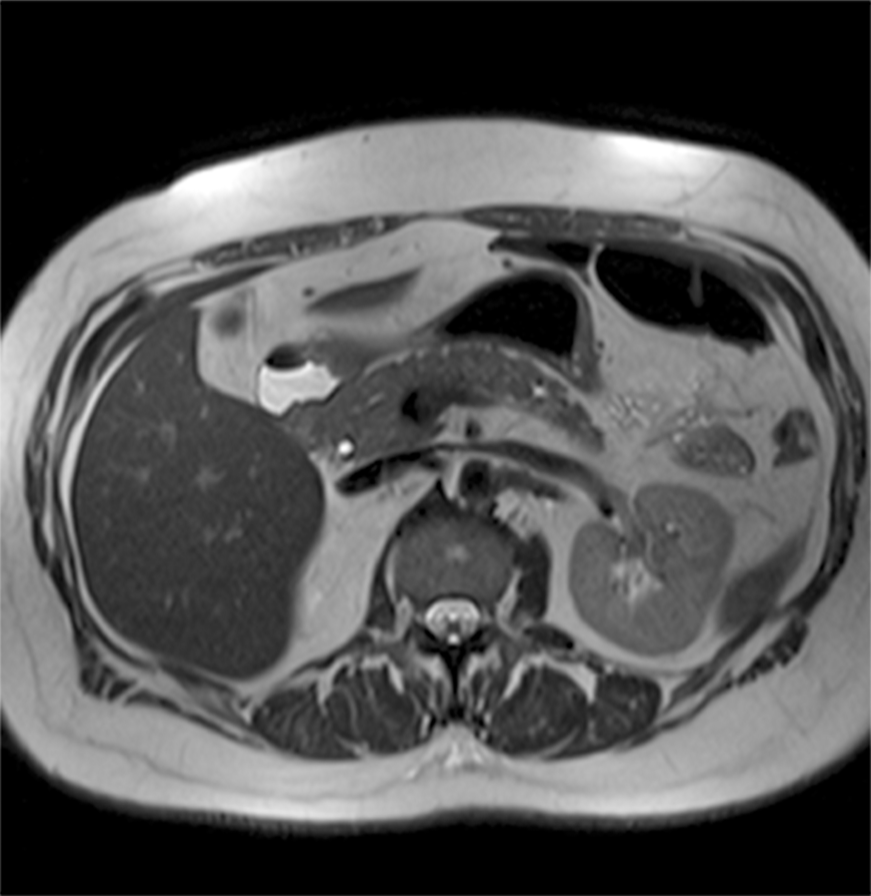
Abdominal MRI (T2-weighted sequence, axial slice), showing an additional small cyst located at the transition between body and tail of pancreas. Same patient as Fig. 2.
